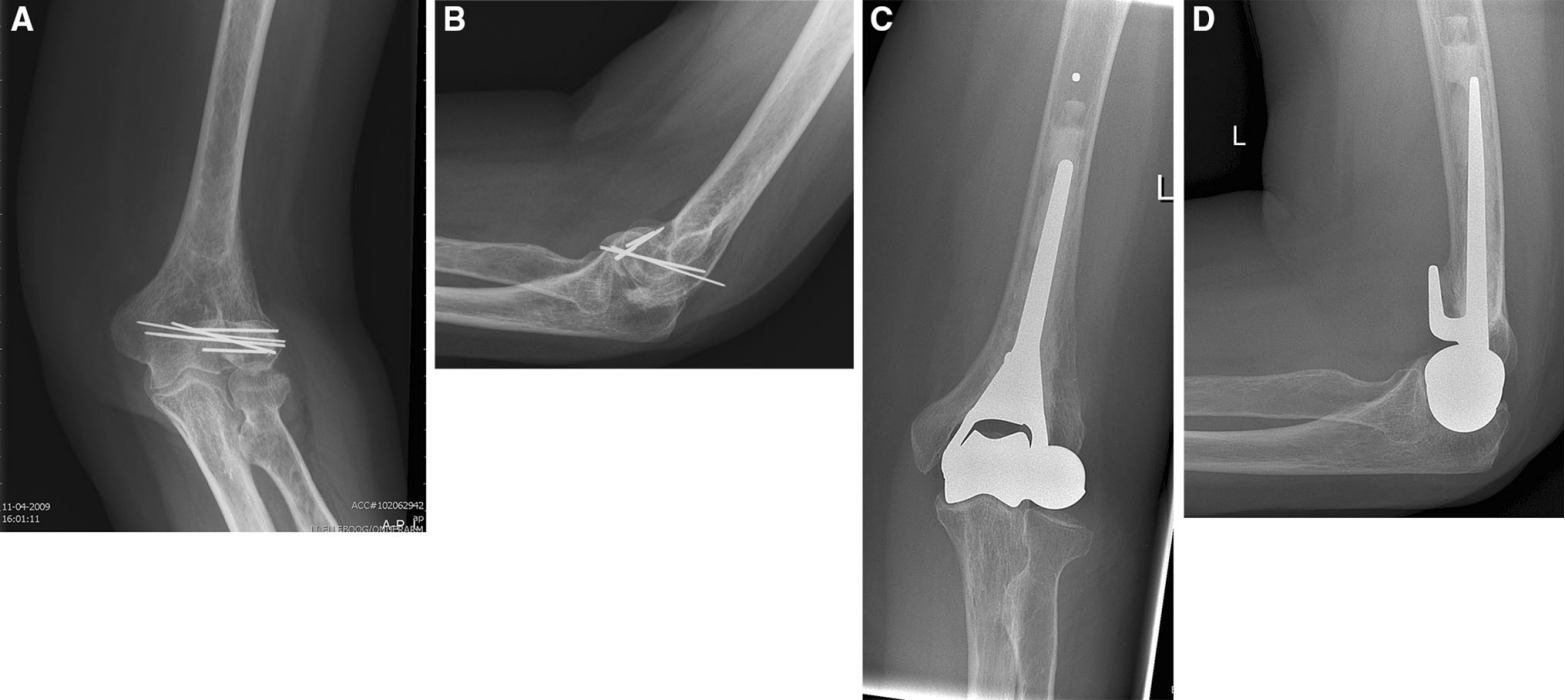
a–d Antero-posterior and lateral radiographic images of the elbow before (a, b) and at end follow-up (c, d) 43 months after distal humerus prosthetic hemiarthroplasty (humeral component of Latitude^®^ Total Elbow with anatomical spool) at end follow-up of case 6. The prosthesis is well positioned, without signs of loosening
